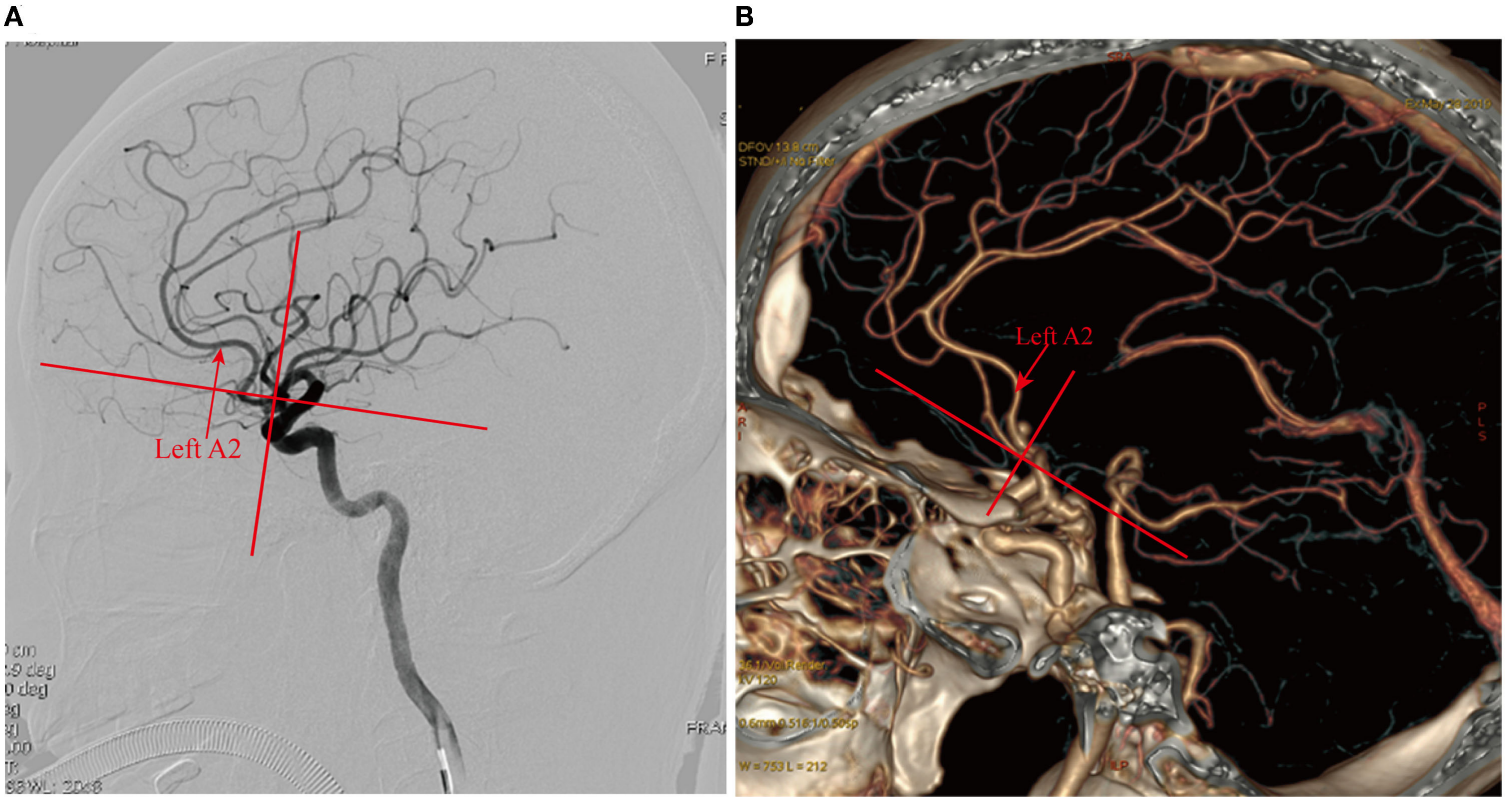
Selection of the operative side based on the anteroposterior relationship of the bilateral A2. (A) DSA after spontaneous subarachnoid hemorrhage showed superiorly projecting microanterior communicating aneurysms, the left A2 located anterior to the right A2, and the left side as the closed side; the right side should be selected as the operative side; (B) CTA examination after spontaneous subarachnoid hemorrhage showed a superiorly projecting AcoA aneurysm, the left A2 posterior to the right A2, and the left side as the open side; the left side should be selected as the operative side.
